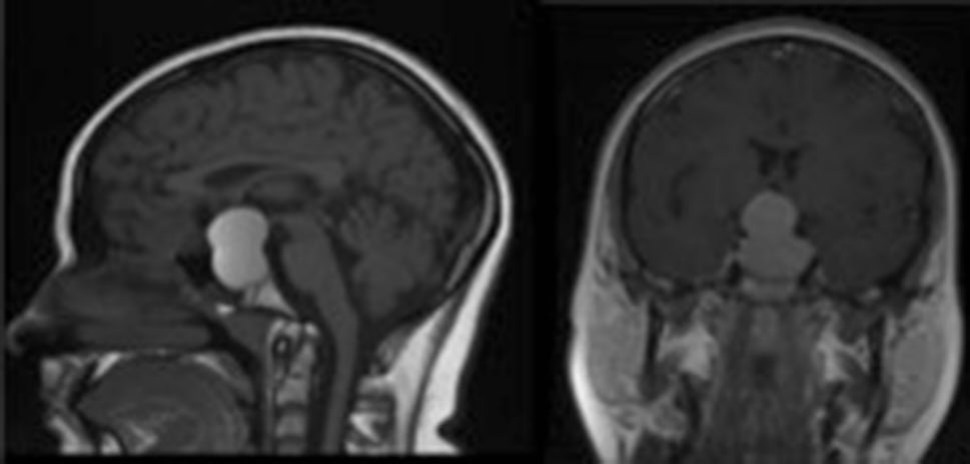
Pre-operative T1 weighted MR image of Child 1 showing a pituitary macroadenoma causing chiasmal compression with extensive high T1 signal within the lesion in keeping with tumour haemorrhage
